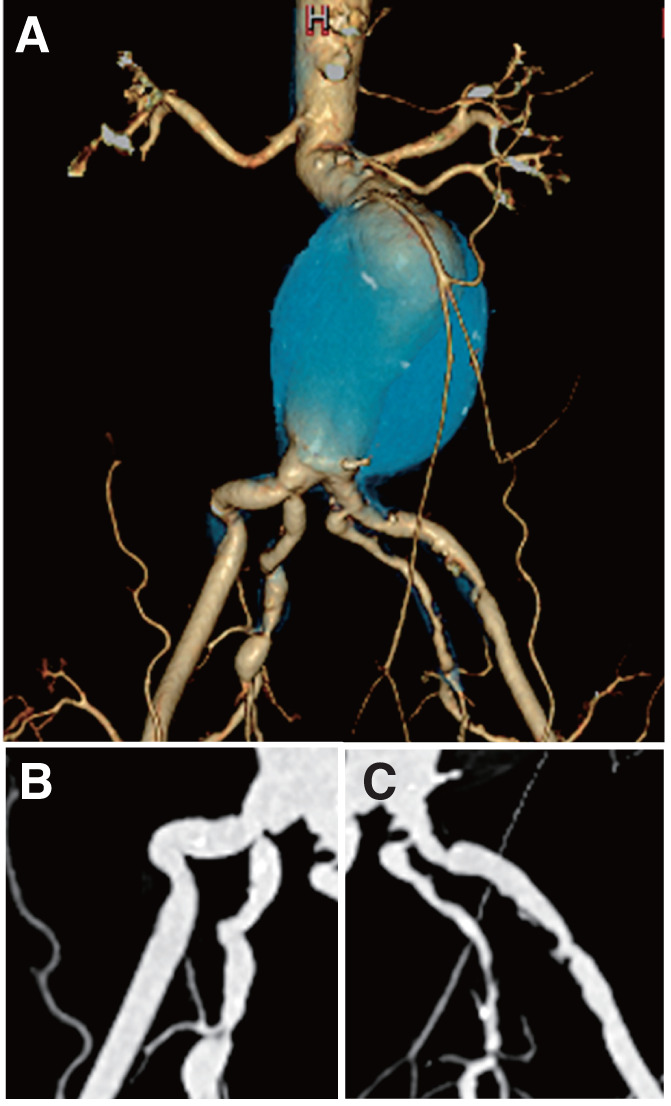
Preoperative computed tomography findings. (A) Three-dimensional reconstructed image showing the extremely short right common iliac artery. (B) Longitudinal view of the right iliac artery showing a short and narrow right common iliac artery (length, 9.6 mm; diameter, 10–12 mm) with severe ostial stenosis of the internal iliac artery (3.2 mm). (C) Longitudinal view of the left iliac artery demonstrating marked hypoplasia and severe ostial stenosis of the internal iliac artery (2.3 mm). These anatomical features precluded the use of iliac branch devices.
